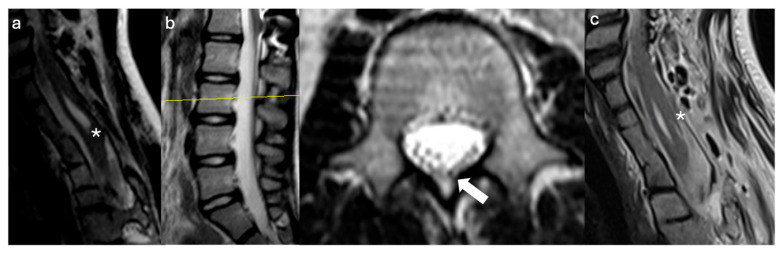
Radiographic Images Case 2: (a) Preoperative sagittal cervicothoracic MRI T2 sequence shows a lower cervical cord syrinx with tethering of the cord to the dorsal dura (asterisk). Note the Klippel–Feil deformity of the cervical spine with incomplete segmentation of the lower cervical vertebra. (b) Preoperative sagittal and axial lumbar MRI T2 sequences show a visible and prominent filum terminale as indicated by the hypointense T2 spot within the cauda equina (yellow bar, arrow, axial T2). (c) Postoperative sagittal cervicothoracic MRI T2 sequence shows decreased syrinx size compared to the preoperative MRI (a) and complete detethering of the previously tethered spinal cord segment and established CSF space after expansile duraplasty (asterisk).
